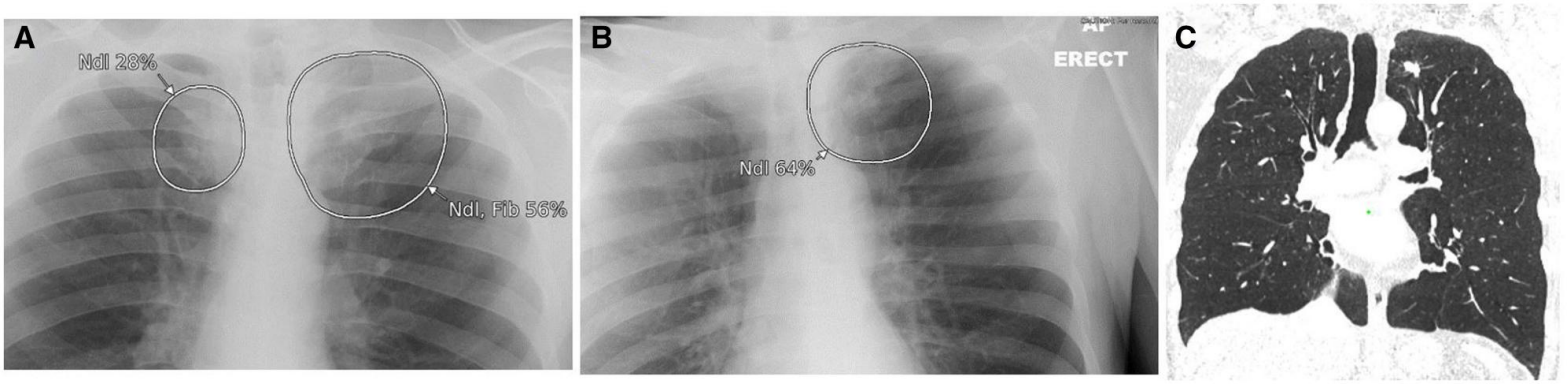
A 72-year-old male smoker with a history of COPD and a recent cough underwent a CXR (A), reported as NAD. The AI software demonstrates suspicion of bilateral nodules. Five months later, the patient returns for a subsequent CXR (B), again reported as NAD. The AI software continues to be suspicious of a nodule in the upper left lobe. Nine months later (14 month from image A), the patient underwent a CT scan (C) for weight loss and change of bowel habit, demonstrating a small left apical mass later confirmed as stage 3a LC. AI = artificial intelligence; CXR = chest radiograph; LC = lung cancer; NAD = no abnormility detected.
